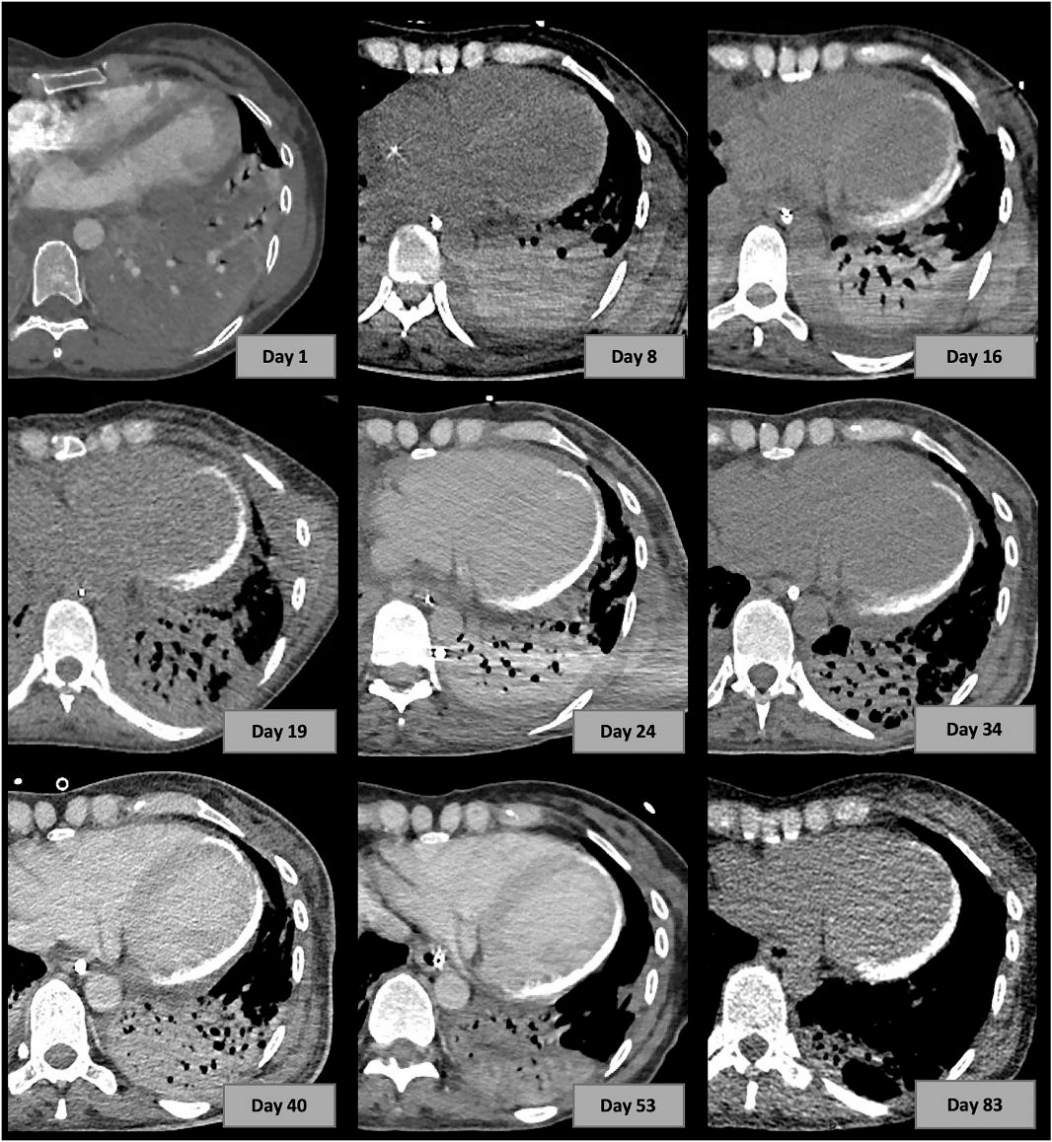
Evolution in myocardial calcification on the serial chest computed tomography scans performed over the time course of the acute respiratory distress syndrome.
